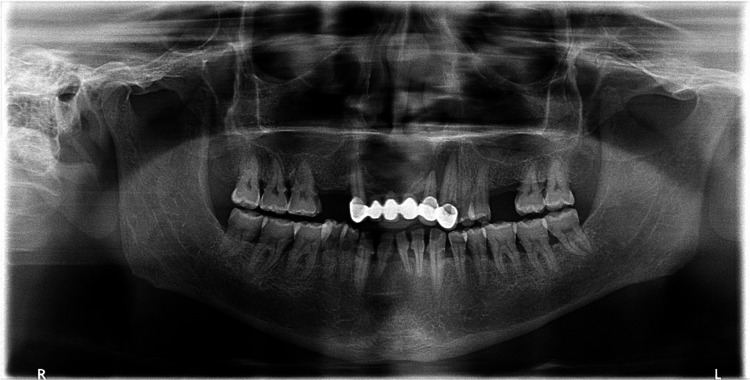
Orthopantomogram showing a heart-shaped lesion in the anterior maxilla
